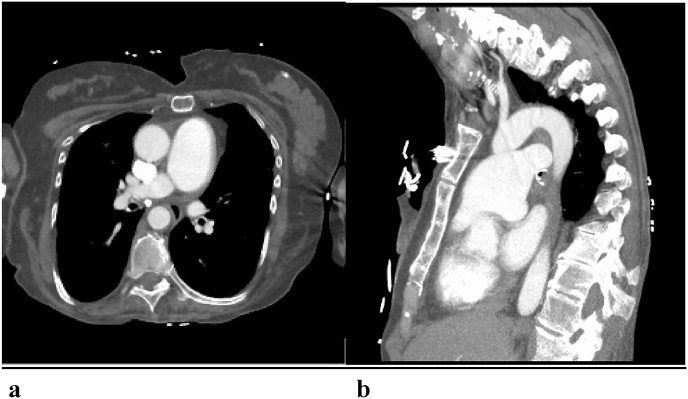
a (left): Repeat serial CTA of the chest at 6-month follow-up demonstrating a 15% reduction in size of the PAA. Fig. 2b: (right): Lateral view of repeat CT Chest at 6-month follow-up demonstrating a 15% reduction in size of the PAA.
